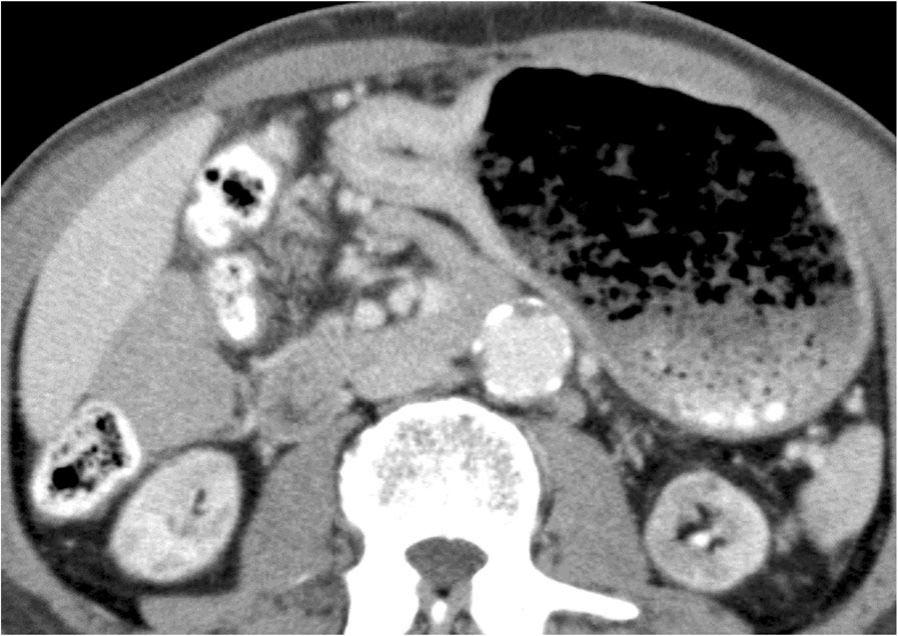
Computed tomography of the abdomen before surgery. Computed tomography of the abdomen revealing focal wall thickening of the prepyloric antrum with several enlarged perigastric lymph nodes
